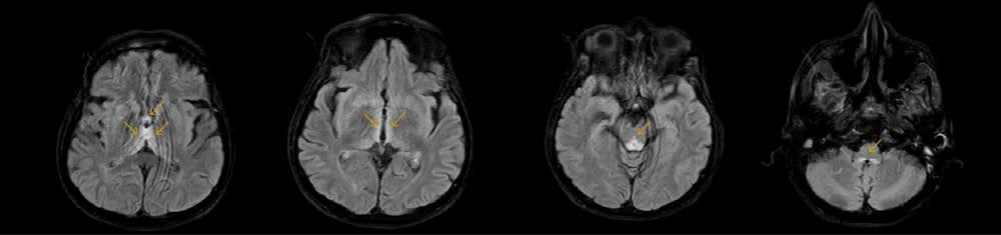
Diffusion-weighted MRI images showing hyperintensities compatible with vasogenic edema observed in the bilateral medial thalamus, mamillary bodies, and dorsal brain stem of the patient in the FLAIR sequences. FLAIR = fluid attenuated inversion recovery, MRI = magnetic resonance imaging.
